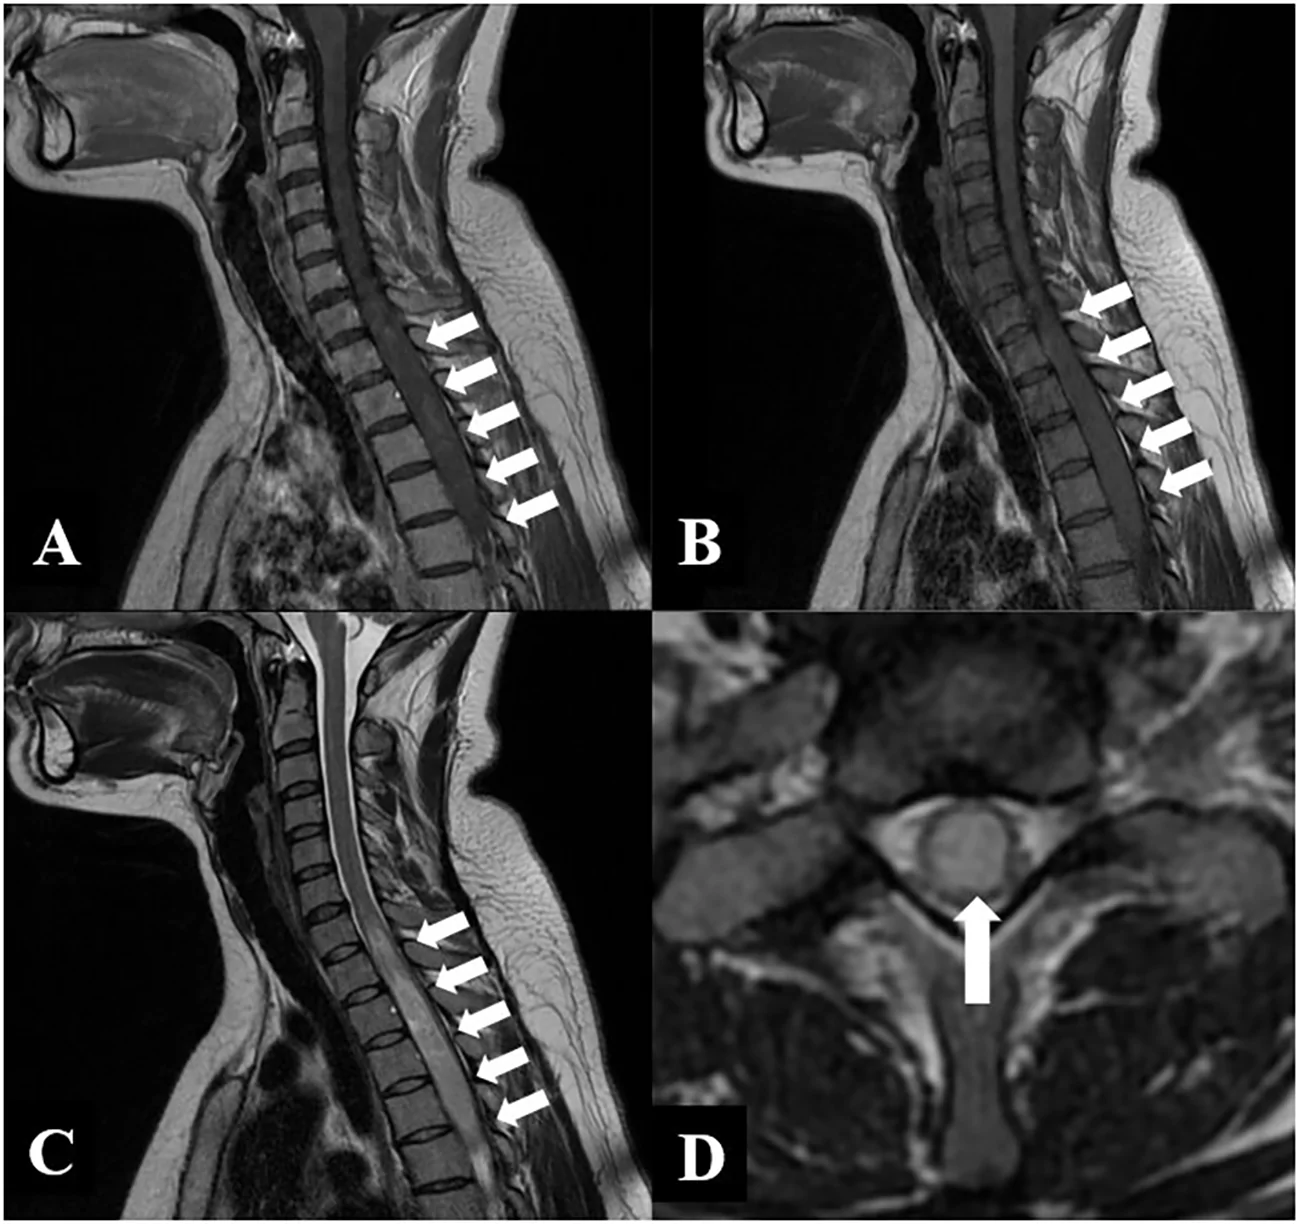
Preoperative magnetic resonance imaging of the cervicothoracic spinal cord. (A) Sagittal contrast-enhanced T1-weighted image; (B) Sagittal T1-weighted image; (C) Sagittal T2-weighted image; (D) Axial contrast-enhanced T1-weighted image. Images demonstrate a centrally located long-segment intramedullary tumour extending from C7 to T5, with fusiform spinal cord expansion and contrast enhancement. White arrows indicate the tumour.
